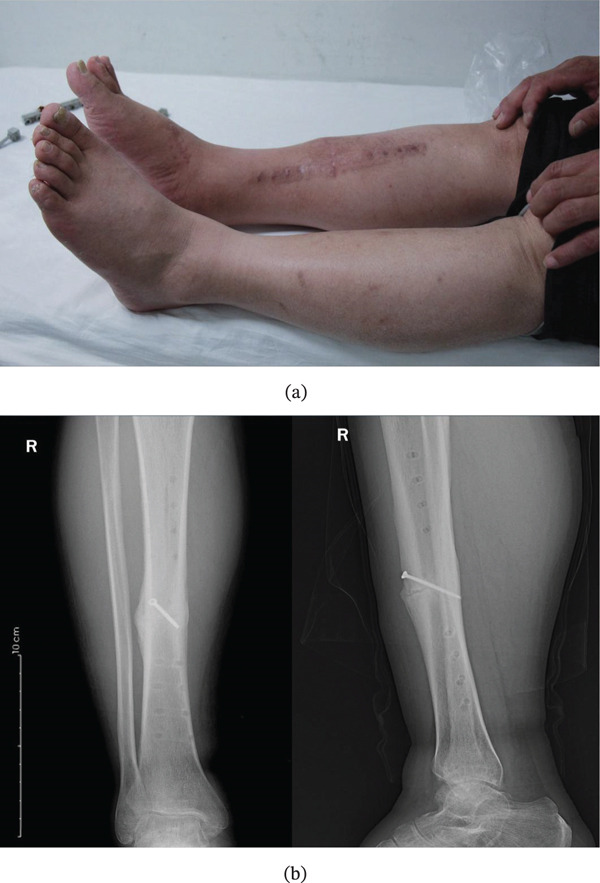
(a) At 6 months postoperatively the American Orthopedic Foot and Ankle Society (AOFAS) score reached 91 points. (b) Anteroposterior (left) and lateral (right) radiographs at the 6‐month follow‐up showing complete bony union with remodeling.
